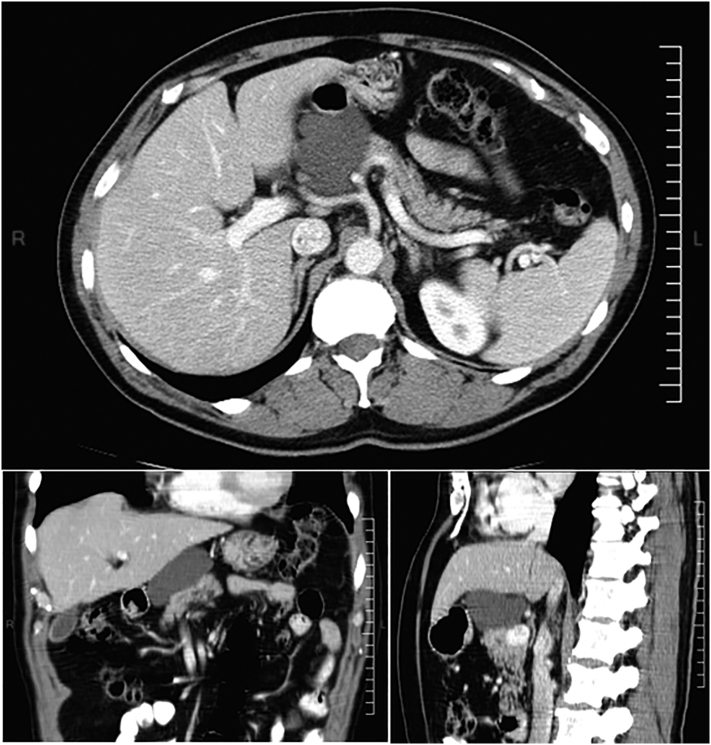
CT abdominal scan (axial, coronal and sagital planes). The nodular cystic lesion located in the head/proximal body of pancreas, with no post contrast enhancement.
